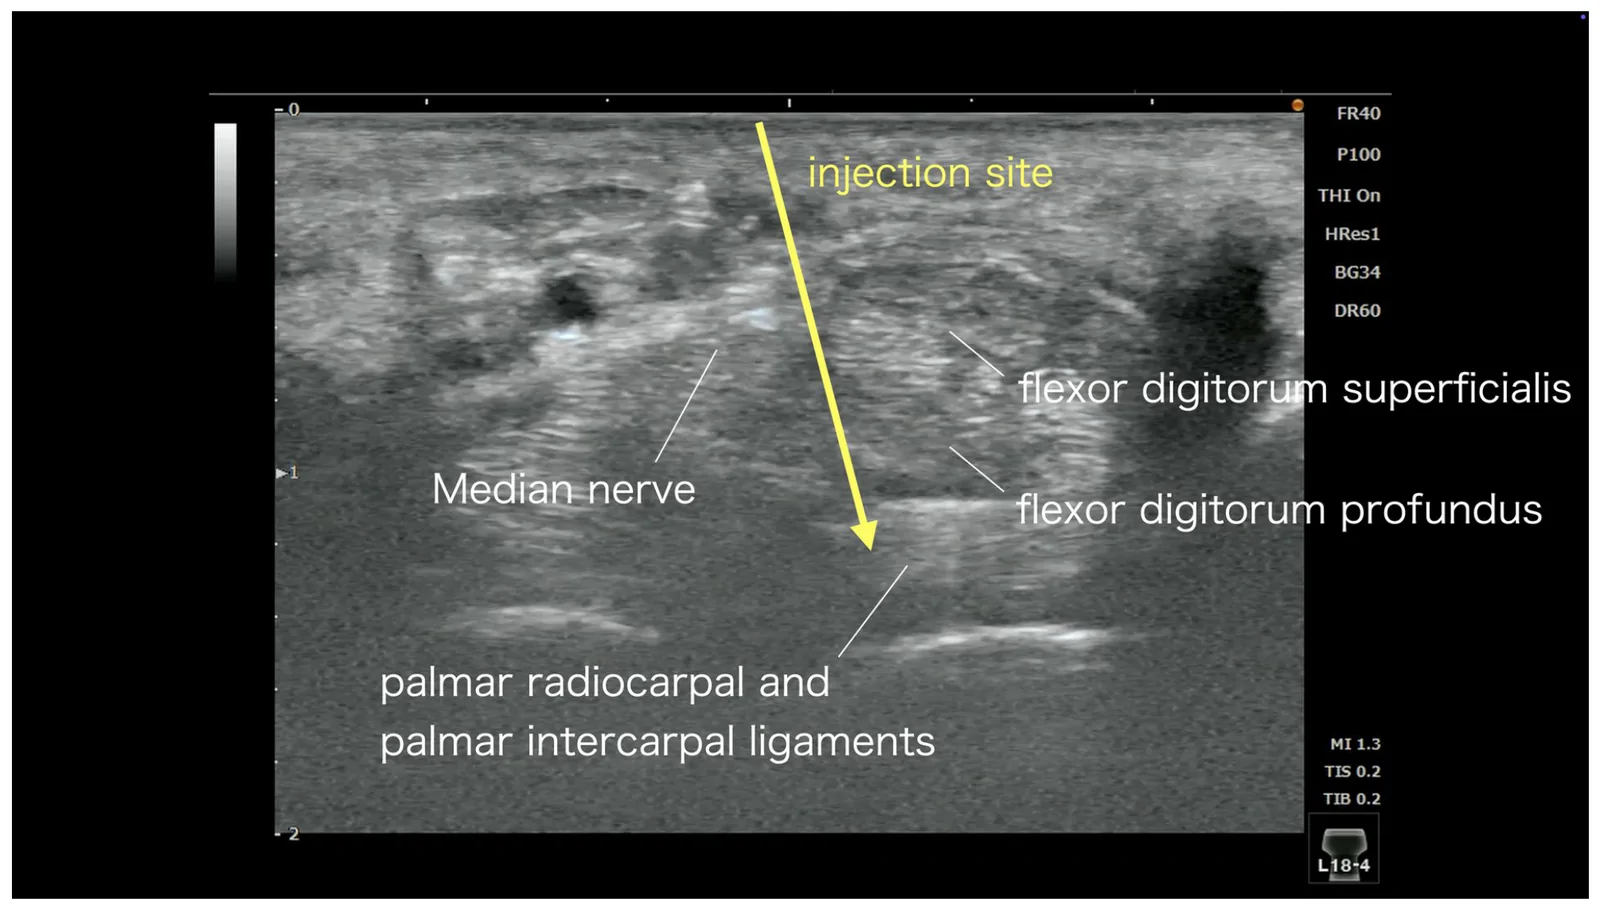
Point 15—palmar radiocarpal and palmar intercarpal ligaments/median nerve: ultrasound-guided fascia hydrorelease; yellow arrow indicates the injection site.
